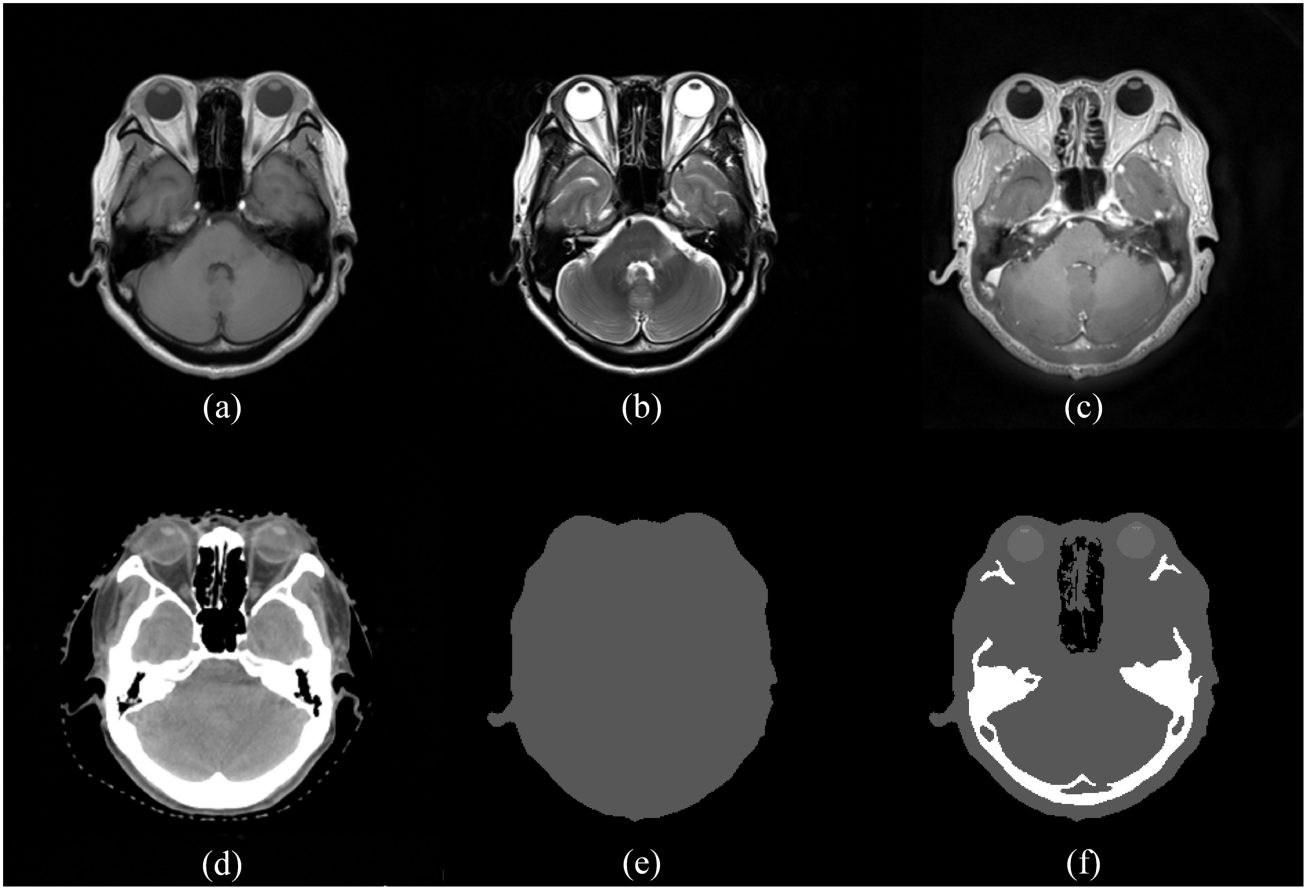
Acquired MR and CT image sets and generated synthetic CT image sets. (a) T1-weighted MR image. (b) T2-weighted MR image. (c) PETRA MR image. (d) Original CT image. (e) Water-equivalent synthetic CT (_W_CT). (f) Bulk-density-assigned CT image (_B_CT).
